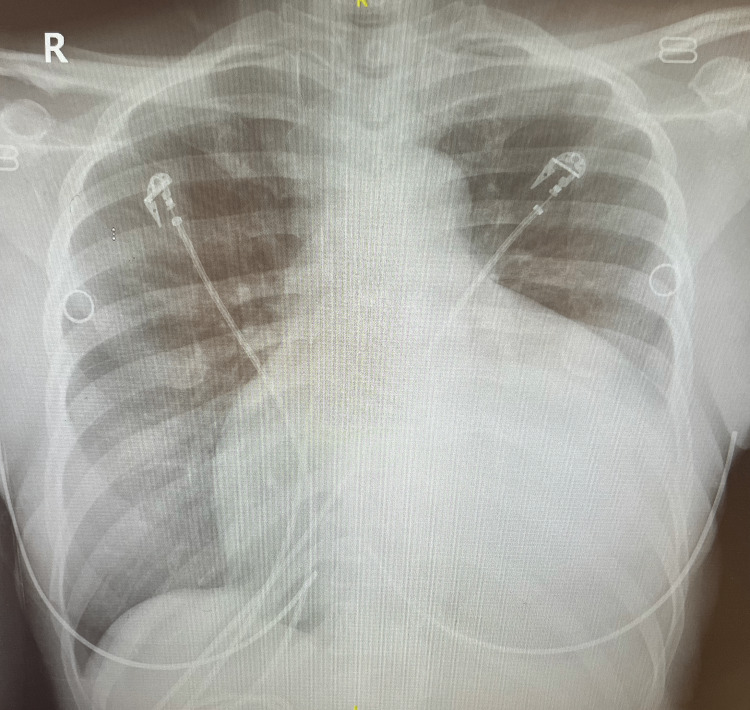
Chest X-ray on admission. Chest X-ray shows significant cardiomegaly with a globular appearance that is compatible with a pericardial effusion.
